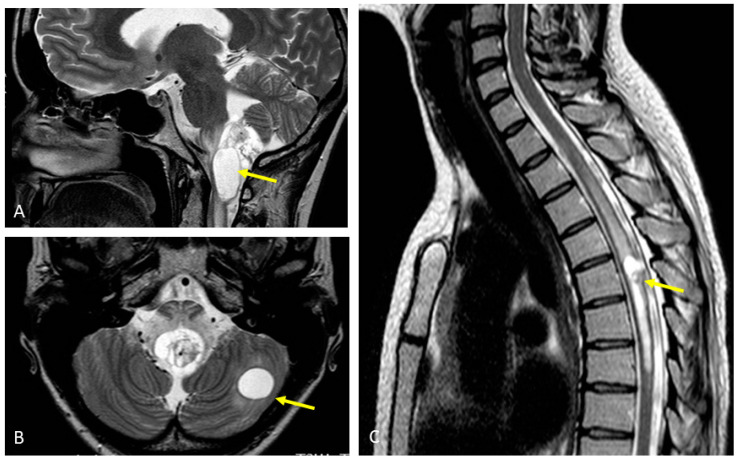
MRI imaging showing CNS lesions of the patient consistent with hemangioblastomas (yellow arrows): (A) Lesion pressing the brainstem and upper spinal cord; (B) Cerebellar lesion; (C) Spinal cord lesion.
